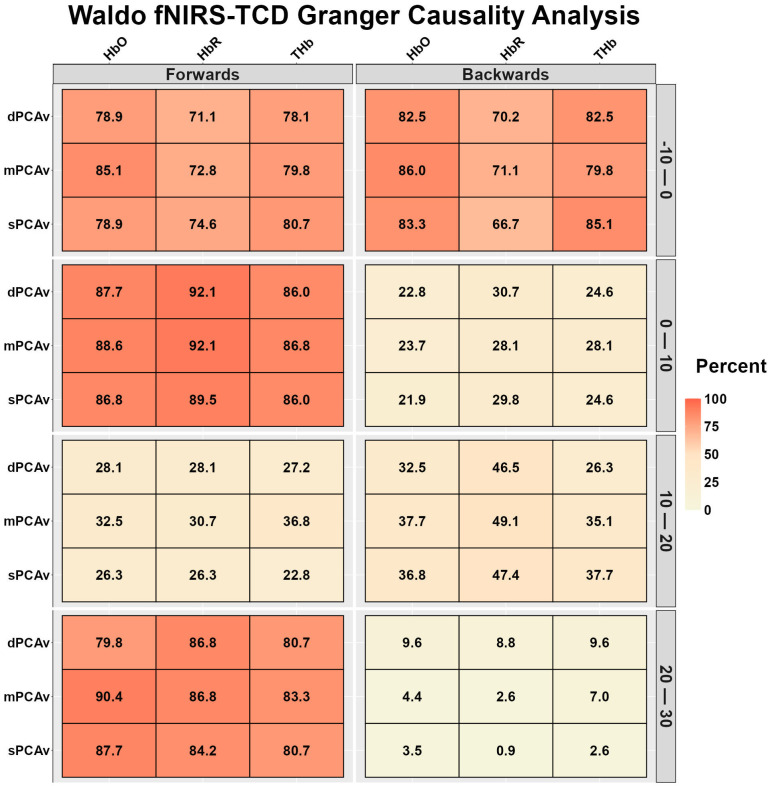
Bidirectional Granger causality analysis results between functional near-infrared spectroscopy (fNIRS) and transcranial Doppler ultrasound (TCD) during the visual “Where’s Waldo?” task. The results are the percentage of participants whose data displayed a significant predictive value between waveforms, stratified into 10-s time bins (*n* = 113, 86 females and 27 males). Oxygenated hemoglobin (HbO), deoxygenated hemoglobin (HbR), and total hemoglobin (THb), diastolic posterior cerebral artery velocity (dPCAv), mean posterior cerebral artery velocity (mPCAv), systolic posterior cerebral artery velocity (sPCAv).
